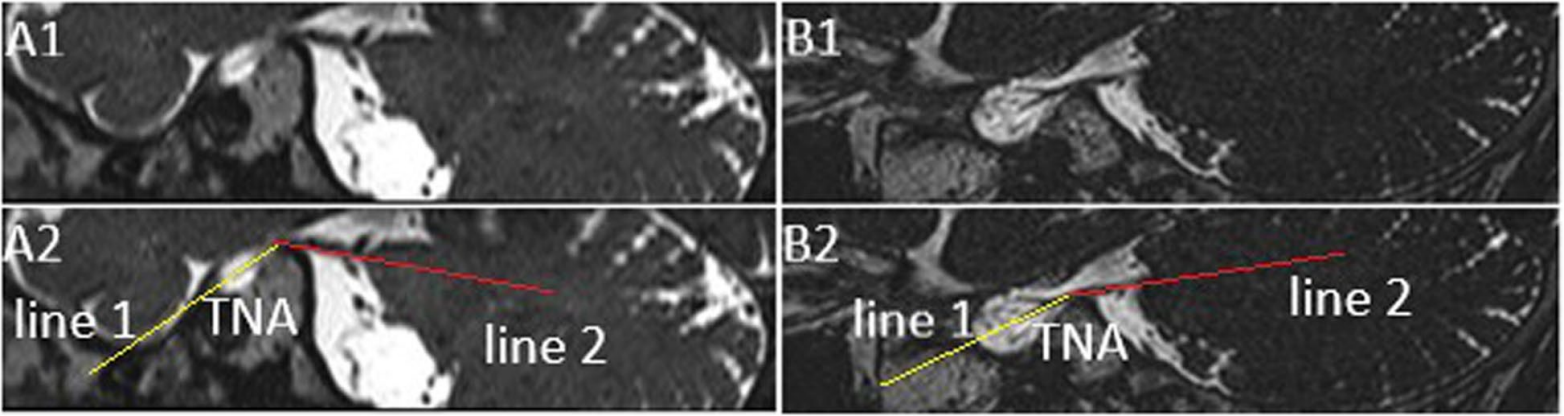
The multiplanar reconstruction of original magnetic resonance image data, 3D-T2-weighted images were reconstructed in the obliquely sagittal plane. The angle between line 1 and line 2 was considered as trigeminal nerve angulation (A2, B2). Color lines represented corresponding lines (yellow: line 1; red: line 2). Line 1: The obliquely sagittal image of trigeminal ganglion (A1-A2). If there were two or more images of the trigeminal branches, the line 1 was the image of the caudal branch of the trigeminal nerve (B1-B2). Line 2: The obliquely sagittal image of the trigeminal nerve on cerebellopontine angle cistern (A2, B2)
